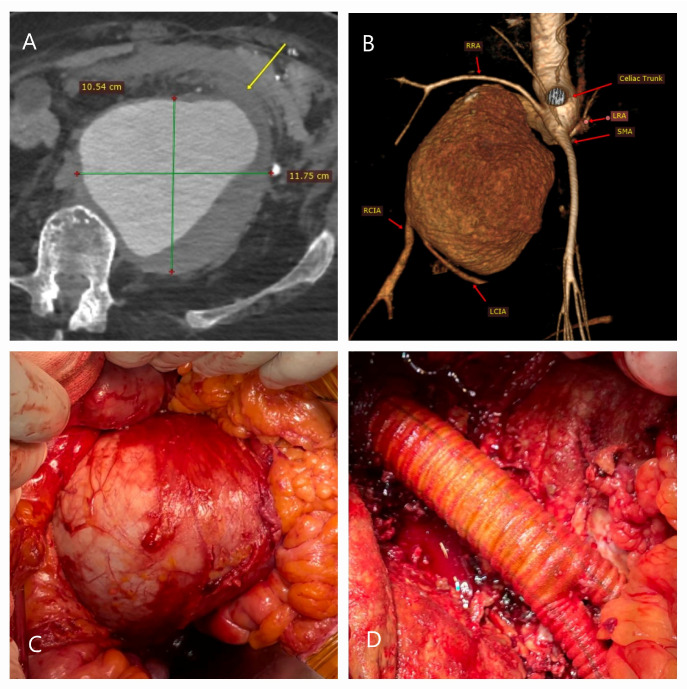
CT and surgical images of a patient with anatomical contraindications for EVAR. A 67-year-old bedridden male with bilateral lower limb amputation and hypertension presented with severe abdominal pain. (A) CT angiography revealed a ruptured aneurysm (11.7 × 10.5 cm) with a probable contained rupture (yellow arrow). (B) 3D CT reconstruction demonstrated hostile neck anatomy and iliac artery configurations. (C) The aneurysm was massive, and (D) after excision, a graft was placed, extending to the femoral arteries due to the inability to perform iliac anastomoses. In such cases, rapid surgical intervention is critical, and adjuncts like autotransfusion, aortic occlusion balloons, or Foley catheters may be necessary to ensure hemostasis and improve intraoperative management.
